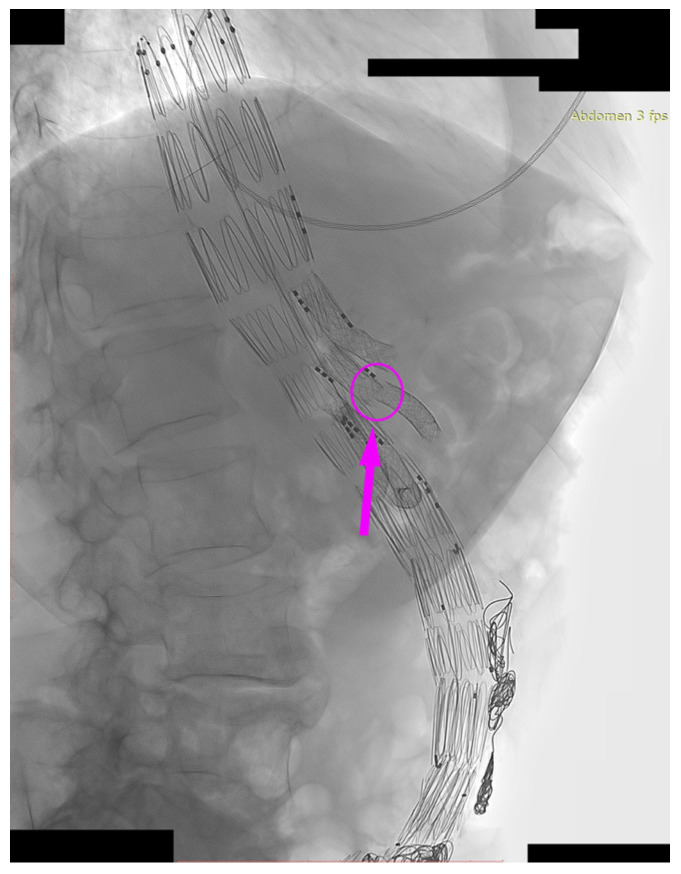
Ruptured superior mesenteric artery branch of stent graft. The fractured site is marked with an arrow. Intraoperative X-ray.
